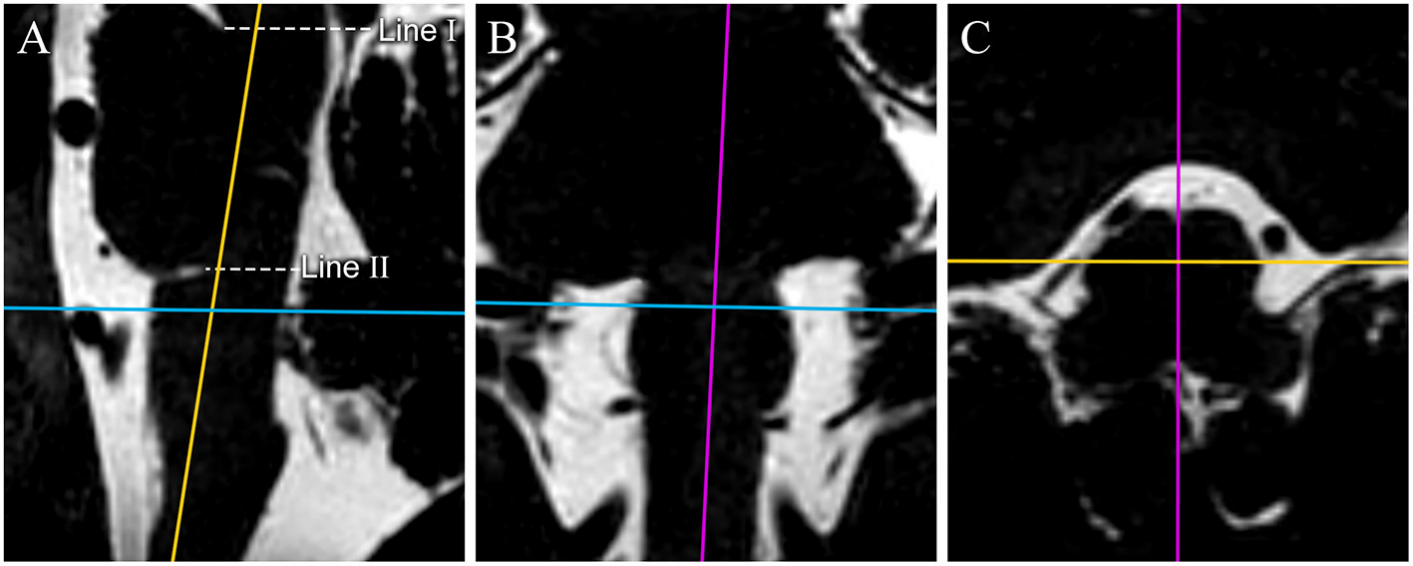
The reconstruction process of original MR image data. 3D-T2-weighted images are reconstructed in the sagittal plane (A), coronal plane (B), and transverse plane (C). Color lines represent corresponding planes (purple: sagittal plane, yellow: coronal plane, and blue: transverse plane). 3D-T2-weighted images are reconstructed to get the median sagittal plane (A) according the anterior median sulcus and posterior median fissure of the medulla oblongata in the transverse plane image (the purple line on C) and the long axis of the medulla oblongata on the coronal plane image (the purple line on B). Line I passes through the superior pontine notch and the inferior edge of the quadrigeminal plate. Line II runs parallel to line I, passes through the inferior pontine notch, and is considered the pontomedullary sulcus. We made a hypothesis in the study: through our adjustment of the plane, the pontomedullary sulcus we confirmed by line II could be on the same horizontal plane. The transverse images are reconstructed based on the auxiliary line on the median sagittal plane image for surveying the CN IX-X REZ.
